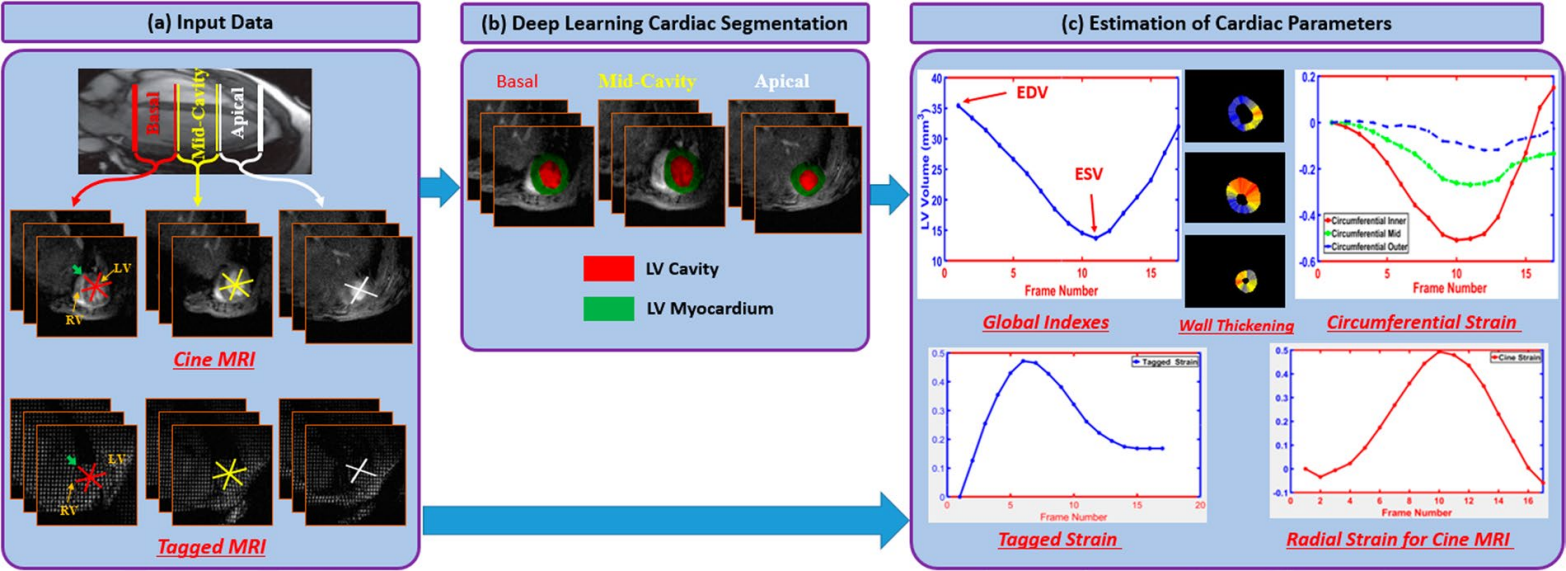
The proposed framework for the analysis of cardiac MRI data.
